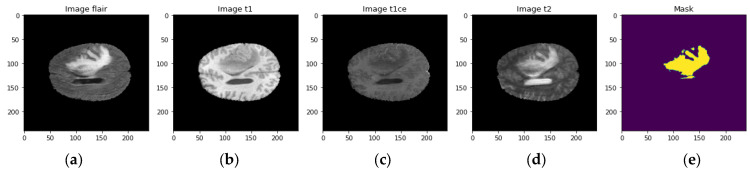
MRI Modalities (a) FLAIR, (b) t1, (c) t1ce, (d) t2, and (e) mask.
